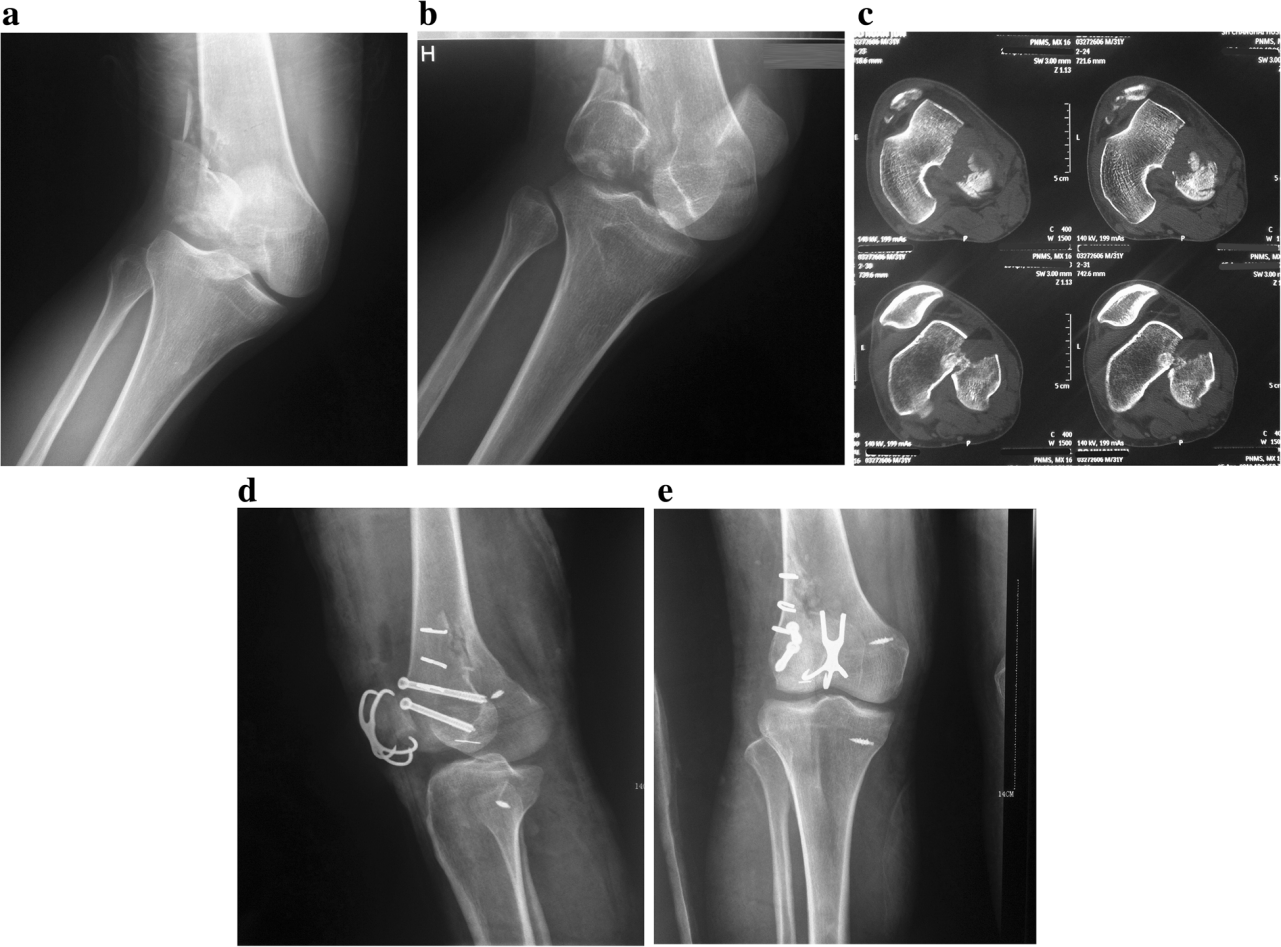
Representative case. (a,b) a 32-year-old male patient was presented with comminuted supracondylar-condylar fracture of the right distal femur, associated with patellar fracture and medial collateral ligament injury. (c) The CT shows coronal plane fracture of supracondylar-condylar femur. (d,e) Post-operation radiographs with 30 months follow-up indicate the fracture has healed well. No evidence of osteonecrosis or development of traumatic arthritis can be observed.
